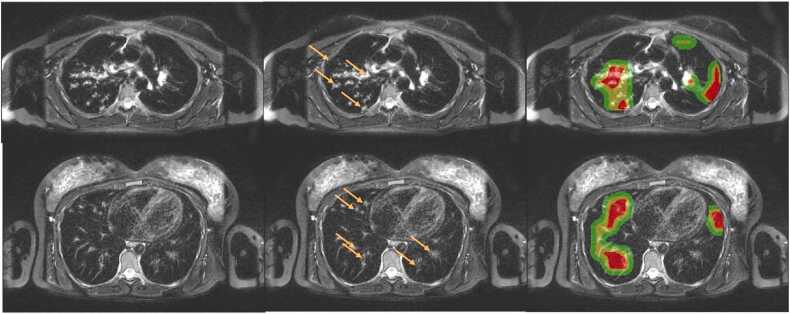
Grad-CAM visualization for mucus plugging. The figure shows (from left to right): the original MRI, the MRI with highlights of mucus plugging (orange arrows) and Grad-CAM an overlay displaying Grad-CAM. Grad-CAM relevance is color-coded, with red indicating highest relevance, yellow moderate relevance, and green lower relevance.
